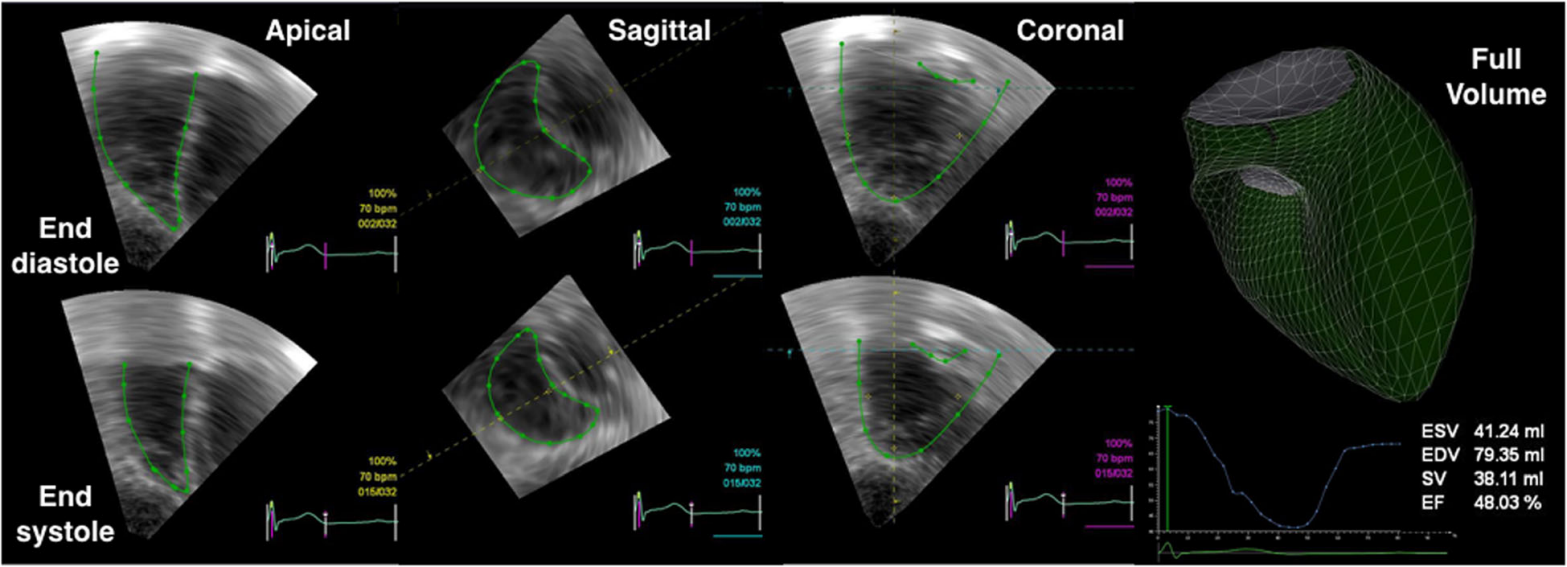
Real-time 3D right ventricular (RV) volume estimation with transthoracic echocardiography. *EDV* End-diastolic volume, *EF* Ejection fraction, *ESV* End-systolic volume, *SV* Stroke volume
